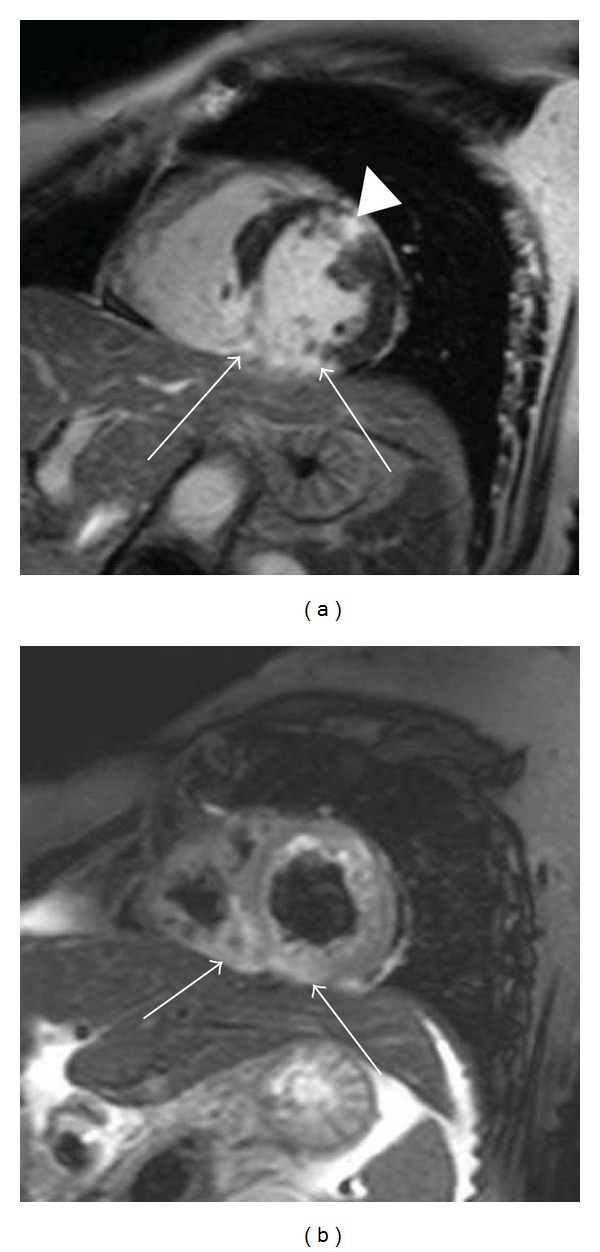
63-year-old female with myocardial infarction. Short-axis delayed-enhancement MRI (a) shows myocardial infarction at the inferior myocardium (arrows) and the anterior myocardium (arrowhead). Short-axis T2-weighted cardiac MRI (b) shows only myocardial edema associated with acute myocardial infarction in the inferior myocardium and right ventricular myocardium (arrows) that are consistent with blood supply from the right coronary artery.
